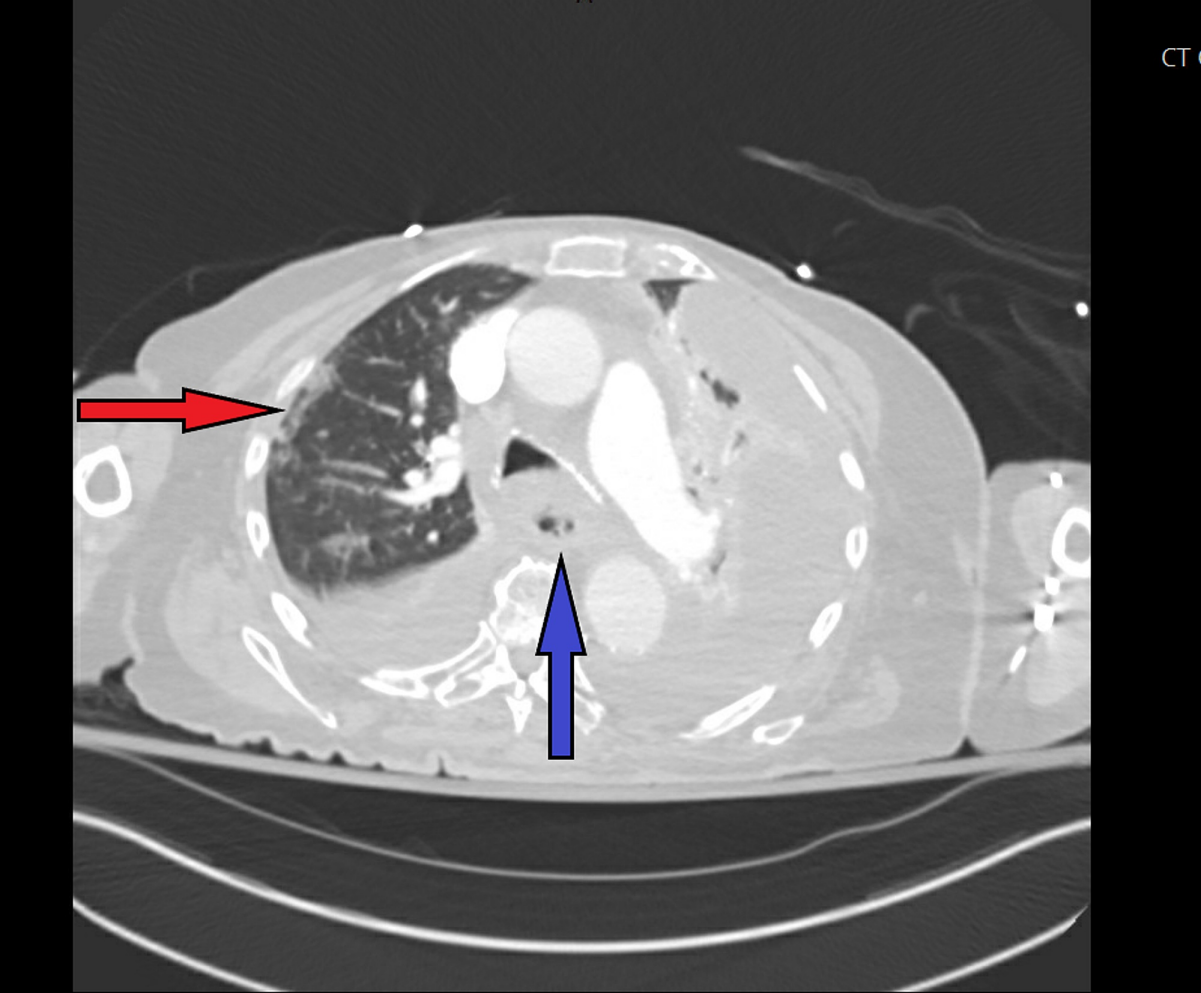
CT angiogram of the chest with multiple pathologic findings Selected image from the patient's CT angiogram of the chest that reveals ground-glass opacities (red arrow) of the right lung field, which is consistent with viral/COVID-19 pneumonia, and an extraluminal air collection (blue arrow) next to the esophagus indicating acute esophageal rupture CT: computed tomography; COVID-19: coronavirus disease 2019
